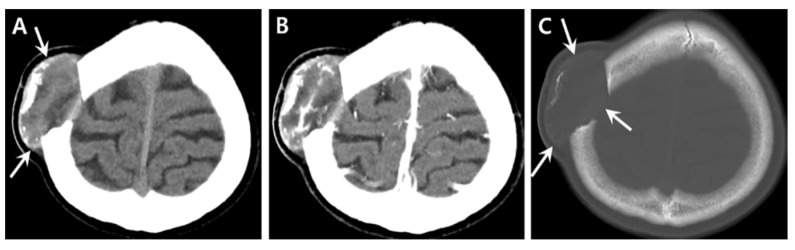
Computed tomography of the brain. (A) Soft tissue window setting. The lesion shows inhomogeneous attenuation with marginal capsule and multifocal bone tissues along the outer margin (arrows). (B) Contrast enhanced axial image showing multifocal septa-like enhancing components within the lesion. (C) Bone window settings. A well-circumscribed, oval, osteolytic lesion is noted in the right parietal bone with multifocal bone tissues in the outer margin, representing the remainder of the outer table (arrows).
